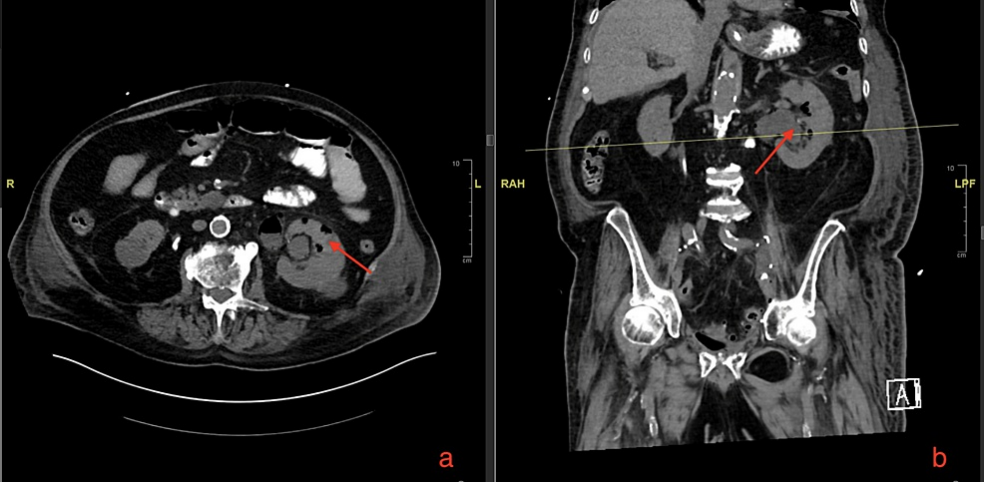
A CT scan of his abdomen pelvis without contrast shows gas in the left renal parenchyma. (a) A CT scan showing the axial section of renal parenchyma with gas. (b) A CT scan showing the coronal section of renal parenchyma with gas.
